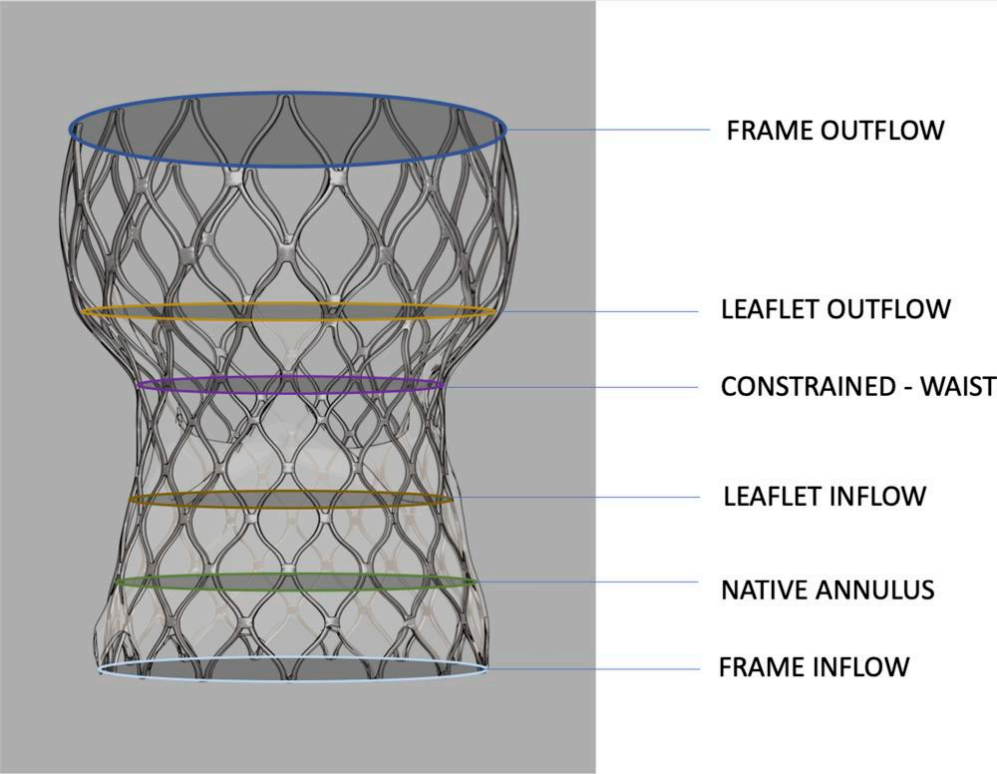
Multi-detector computed tomography measurements of major and minor diameters were performed at six levels of the prosthetic valve (Evolut R, medtronic): (i) frame inflow (nadir of the prosthesis); (ii) native annulus; (iii) leaflet inflow (nadir of the prosthetic leaflets); (iv) prosthesis waist (the waist between the leaflet outflow and the leaflet inflow levels); (v) leaflet outflow (the three commissural tabs of the prosthetic leaflets); (vi) frame outflow (tip of the prosthesis).
